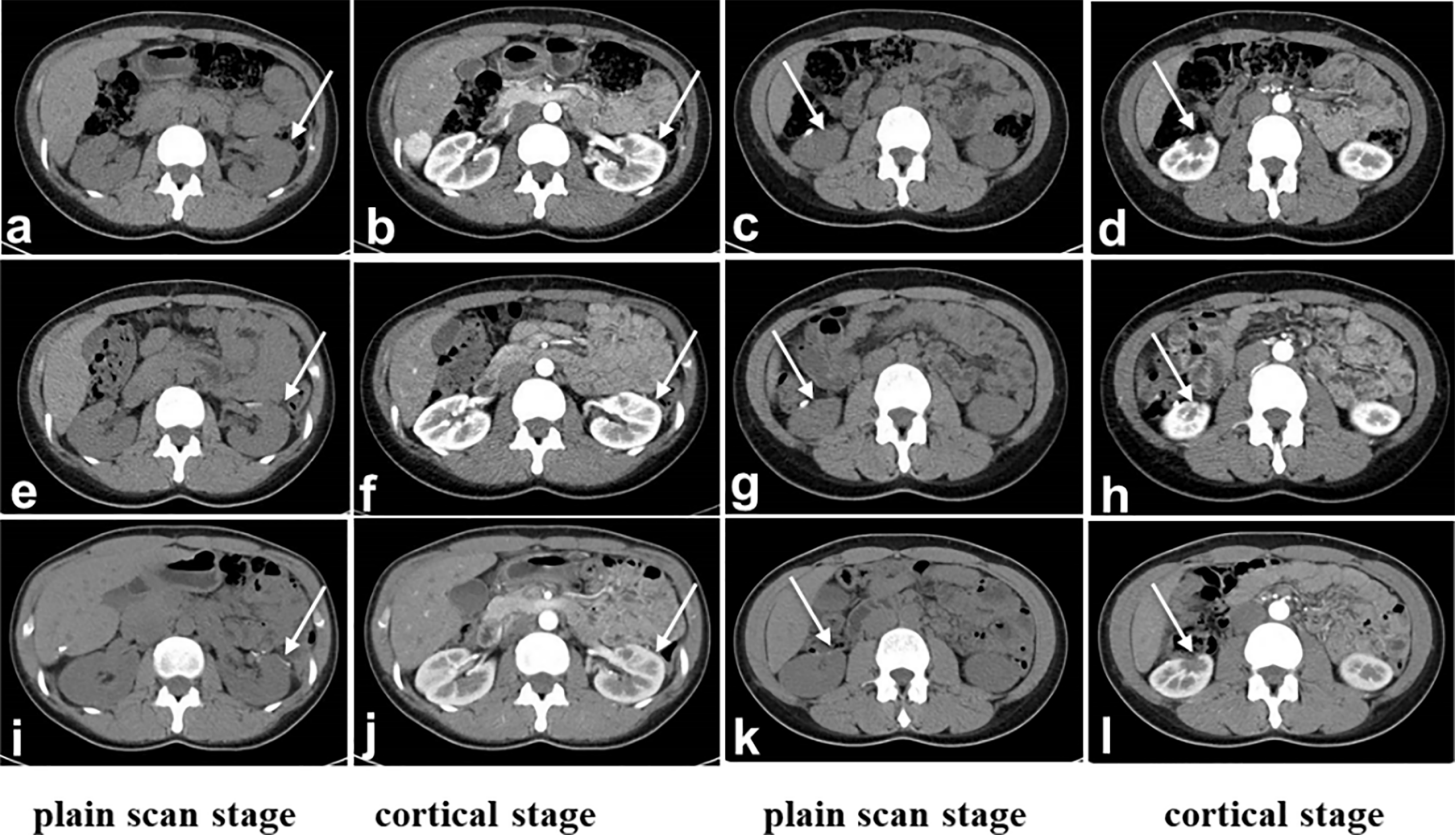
CT after surgery. (A-D): Third month after surgery, (E-H): 6 months after surgery, (I-L): 24th month after surgery. The margins of the upper left kidney (A, B, E, F, I, J) and lower right kidney (C, D, G, H, K, L) were irregular. The perirenal space of the bilateral kidneys was clear, and the shape and size were normal. There was no obvious enlarged lymph node shadow in the retroperitoneum. (A, E, I): Plain scan stage. (B, F, J): Cortical stage. (C, G, K): parenchymal stage. (D, H, L): Renal pelvis stage. The white arrow indicates status of partial nephrectomy.
